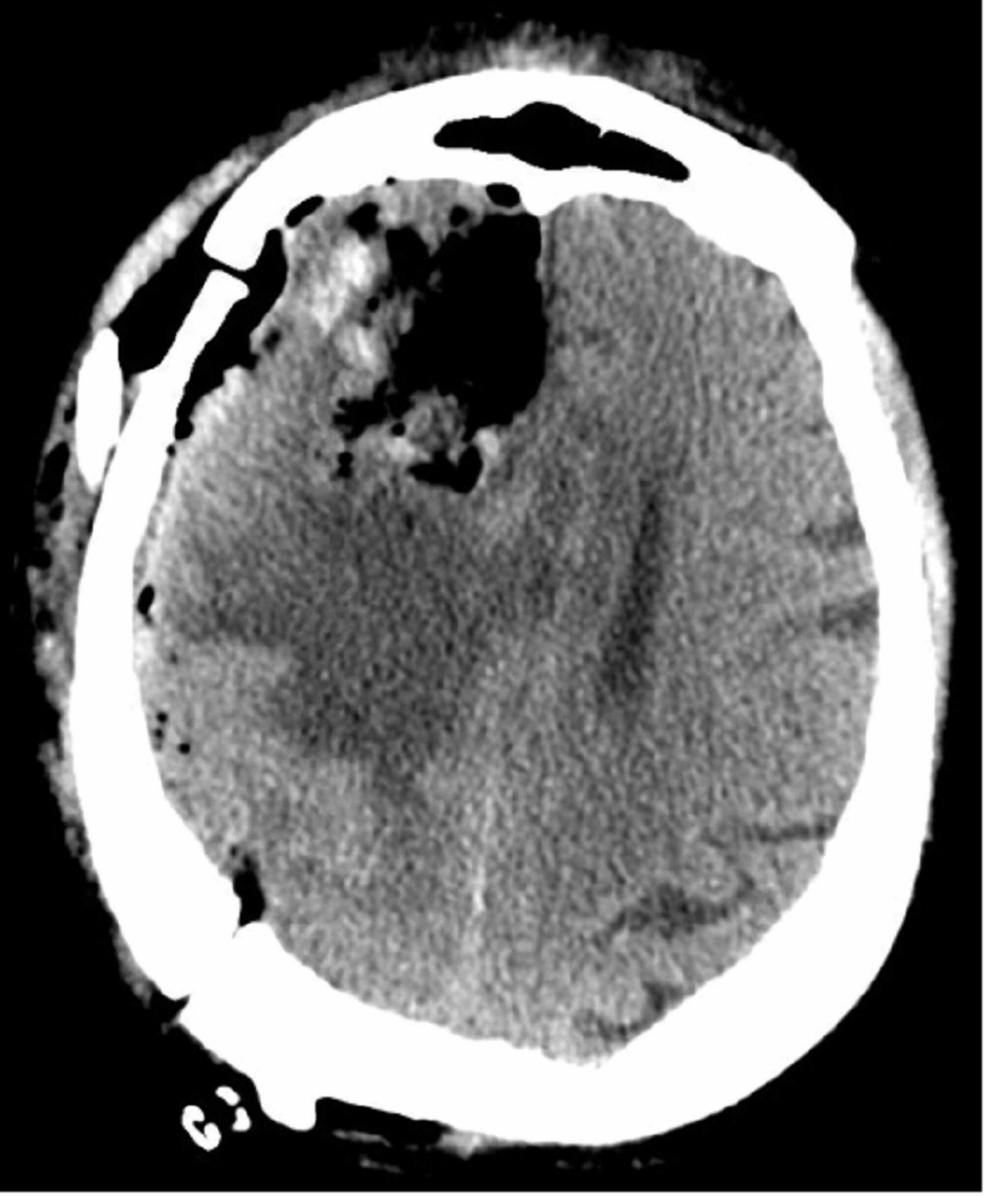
Post-operative non-contrast CT head Post-operative imaging demonstrates expected surgical change after a right frontal craniotomy with gross total resection of the right lobar hemorrhagic mass and restoration of pre-operative midline shift. Vasogenic edema appears stable.
